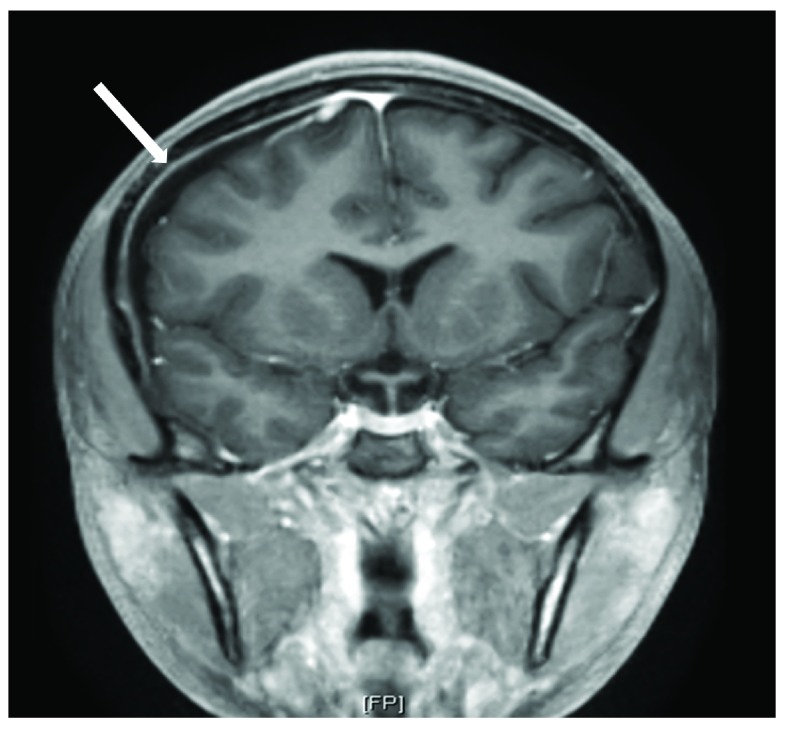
Coronal view of MRI brain post-contrast showing extra-axial collection with the arrow indicating dural enhancement.
